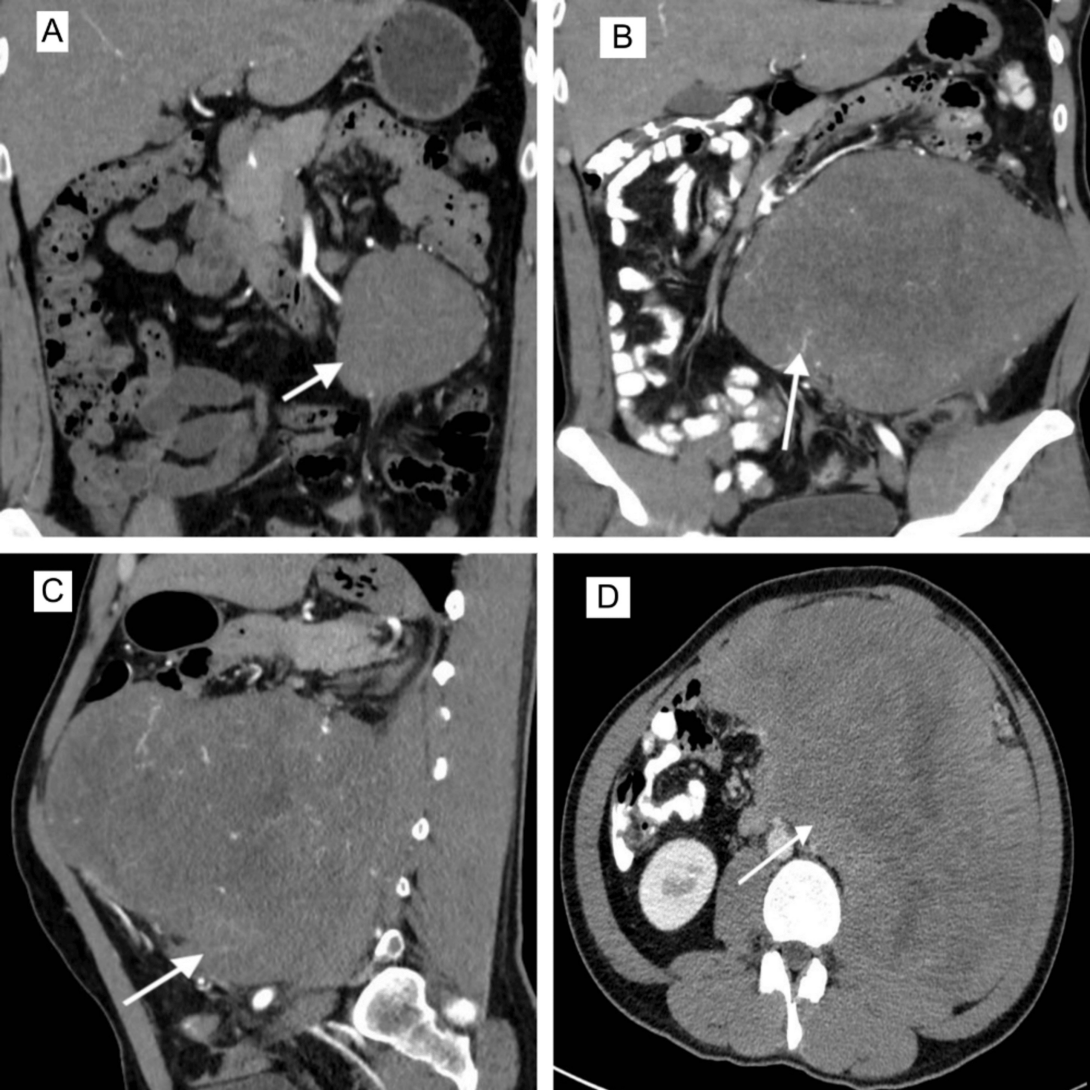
CT scan image of abdomen 1A (Coronal view)—Arrow showing retroperitoneal tumor on initial presentation; 1B (Coronal view), 1C (Sagittal view), 1D (Axial view)—Arrows showing large retroperitoneal tumor after second presentation
